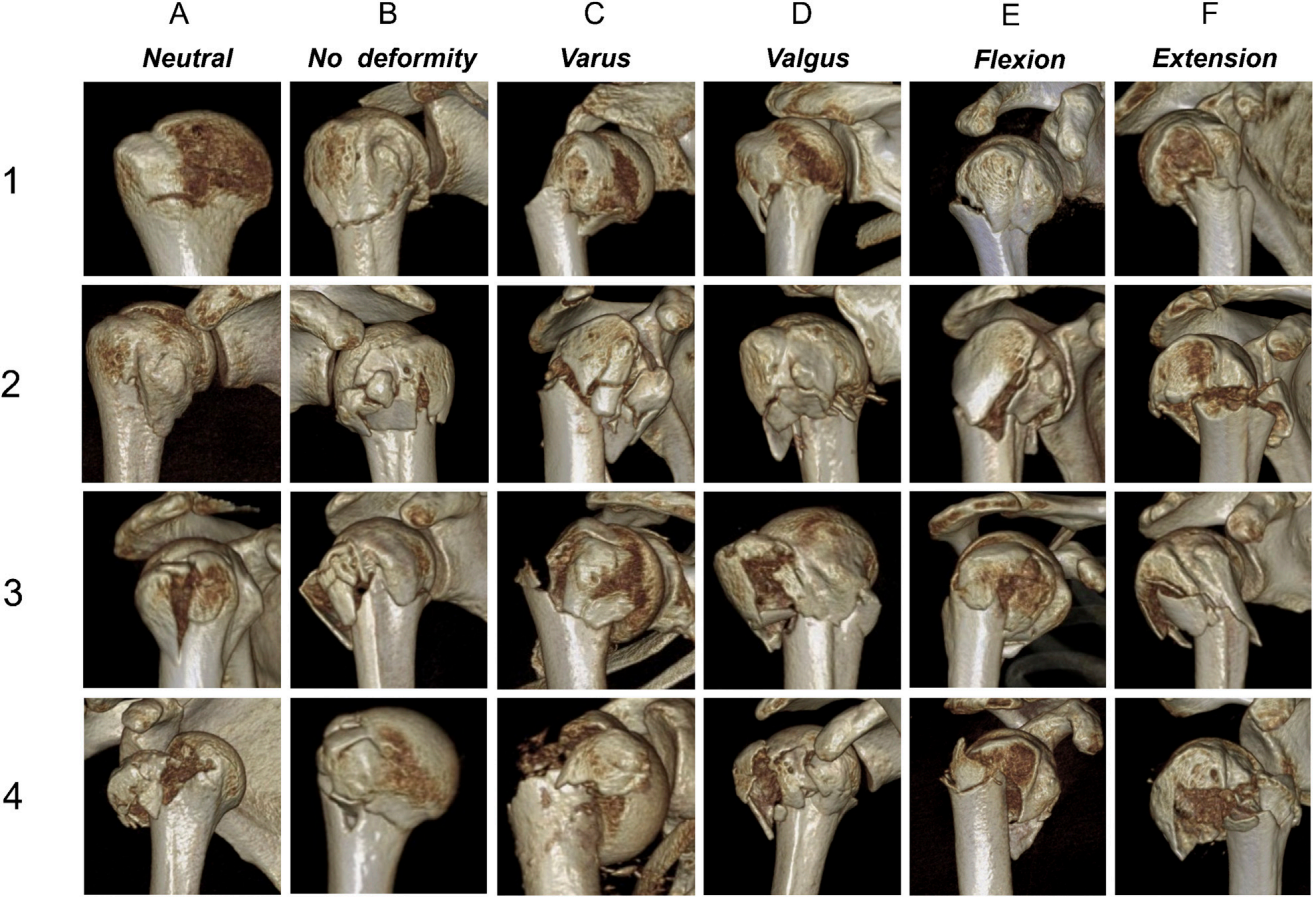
Three-dimensional computed tomography examples for each fracture. (1–4) The main classification criteria; (A–F) The secondary classification criteria.
